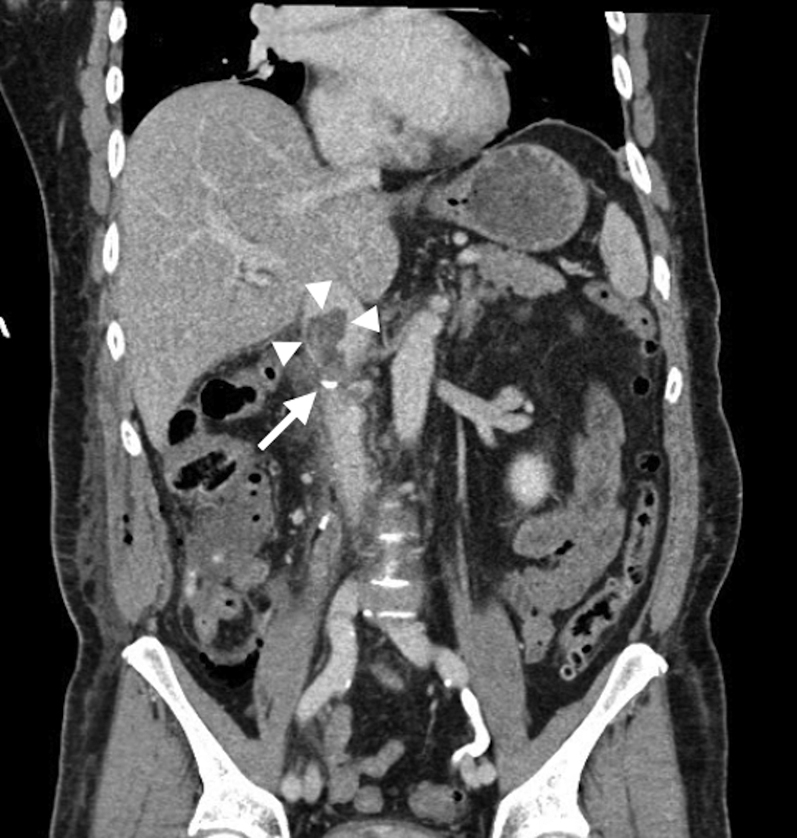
Computed Tomography of Inferior Vena Cava Tumor Thrombus Coronal computed tomography demonstrating inferior vena cava thrombus (arrowheads) adjacent to a nephrectomy staple (arrow).
